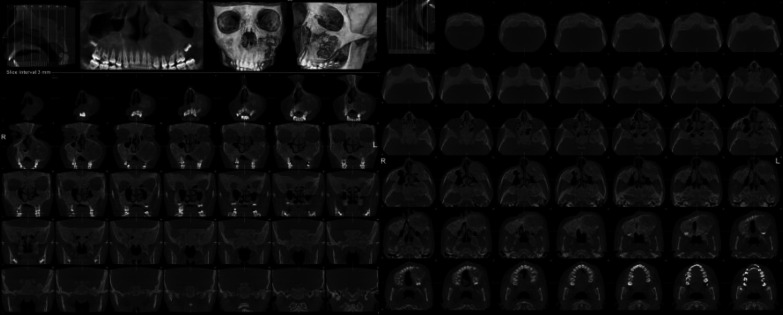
Computed tomography scan of the patient
